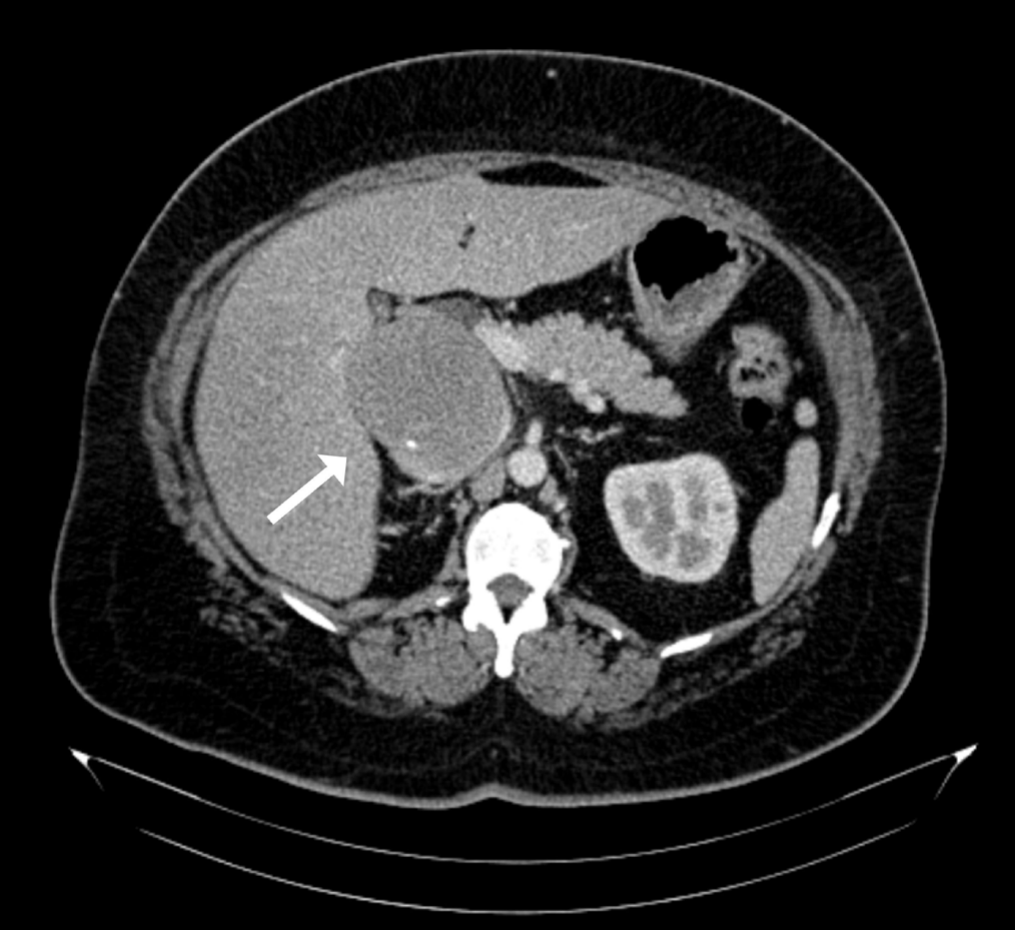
Computed tomography image of the abdomen from the arterial phase, showing the tumor exerting mass effects on the liver and considerable reduction of the lumen of the retro-hepatic vena cava.
